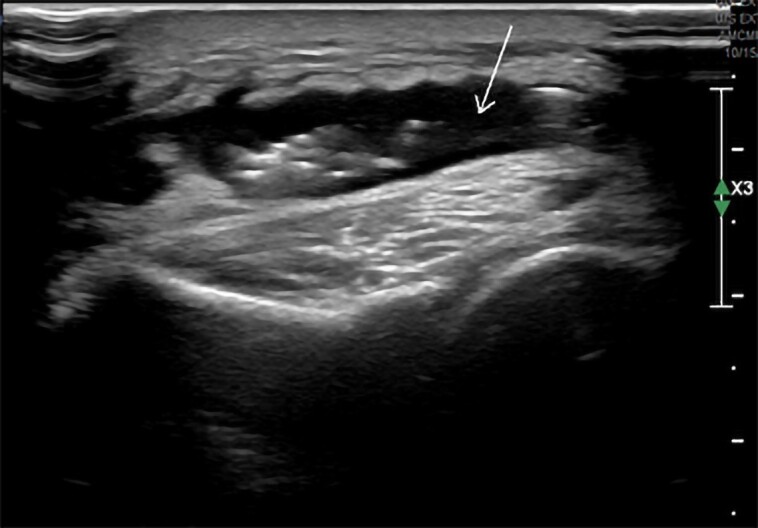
Ultrasound imaging of left olecranon abscess (arrow).
